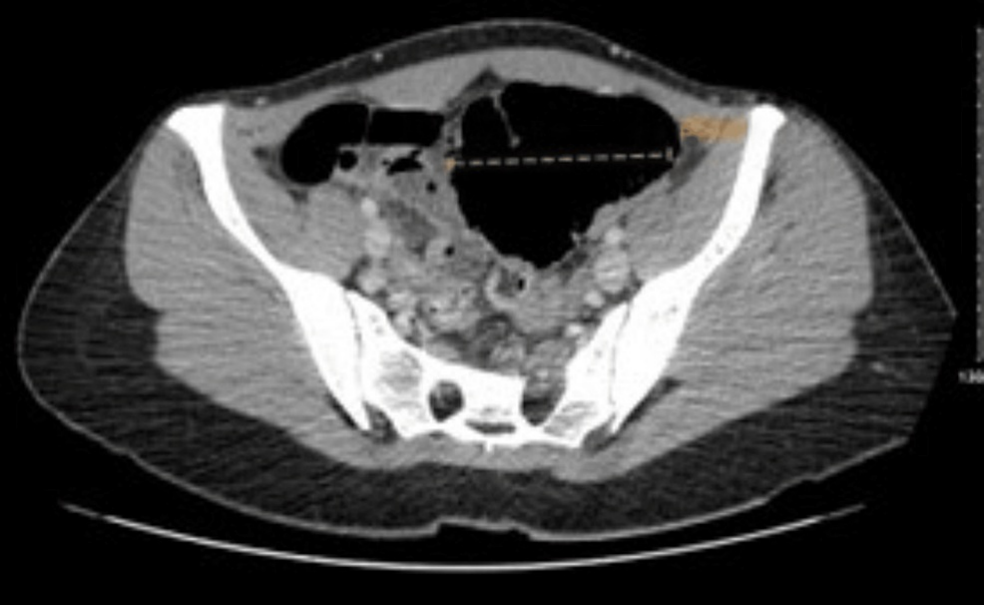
CT scan of abdomen and pelvis with contrast in axial view, showing distended caecum, seen to the left of the midline
